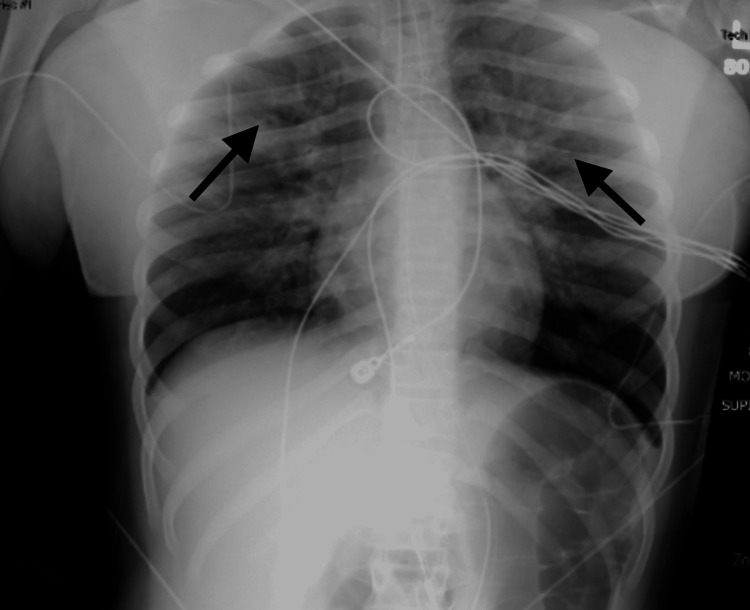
Chest X-ray with arrows demonstrating bilateral airspace opacities indicative of suspected fluid overload. Endotracheal tube in adequate position.
